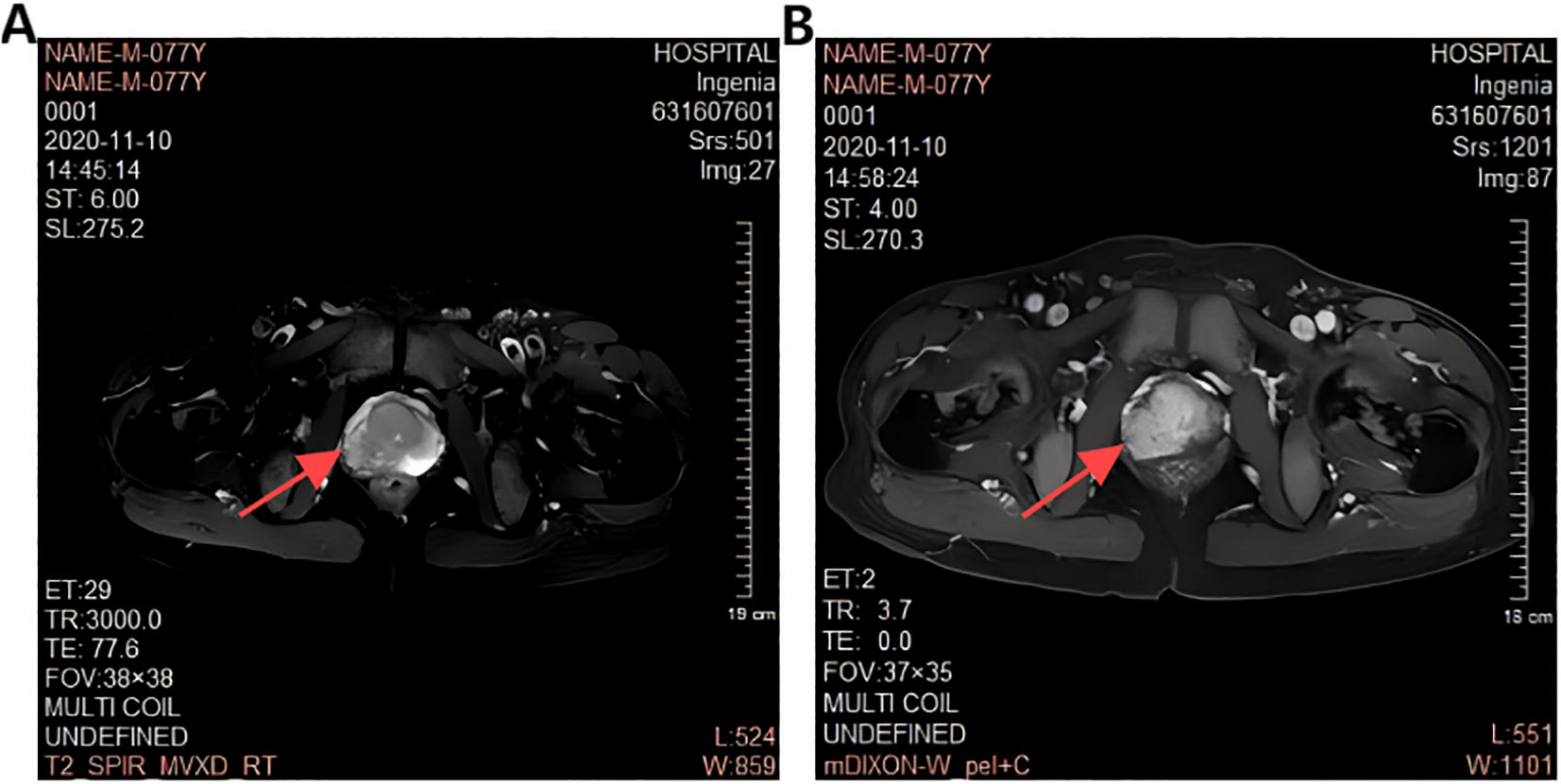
Prostate Magnetic Resonance Examination (A): Unevenly decreased T2W1 signal in the peripheral band (red arrows). (B): Enhanced DWI signal in the peripheral band (red arrows).
